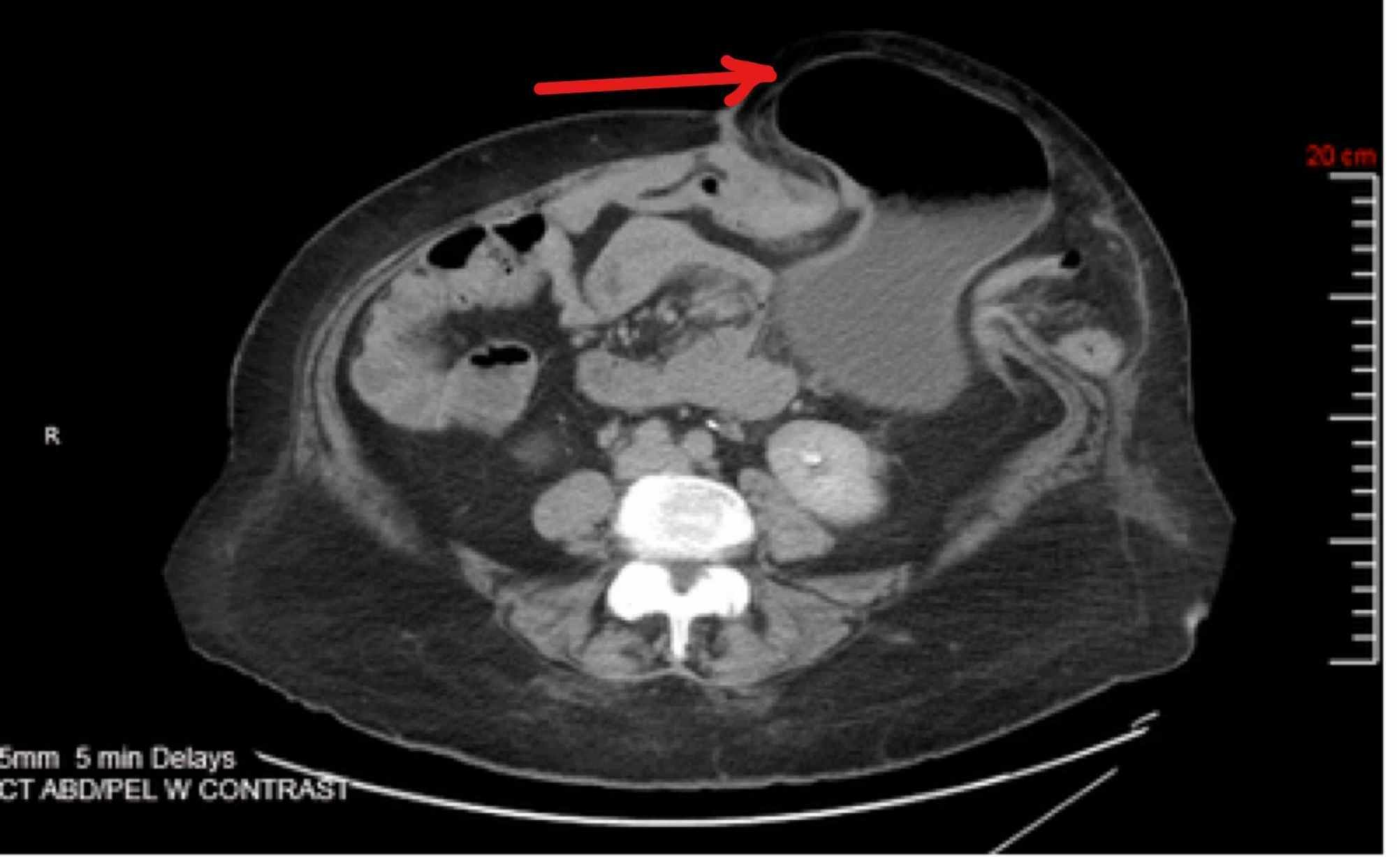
CT abdomen and pelvis CT abdomen demonstrating a large parastomal hernia. There is a portion of the stomach, small bowel, colon, and mesentery within the hernia sac (Arrow indicating the location of the hernia).
